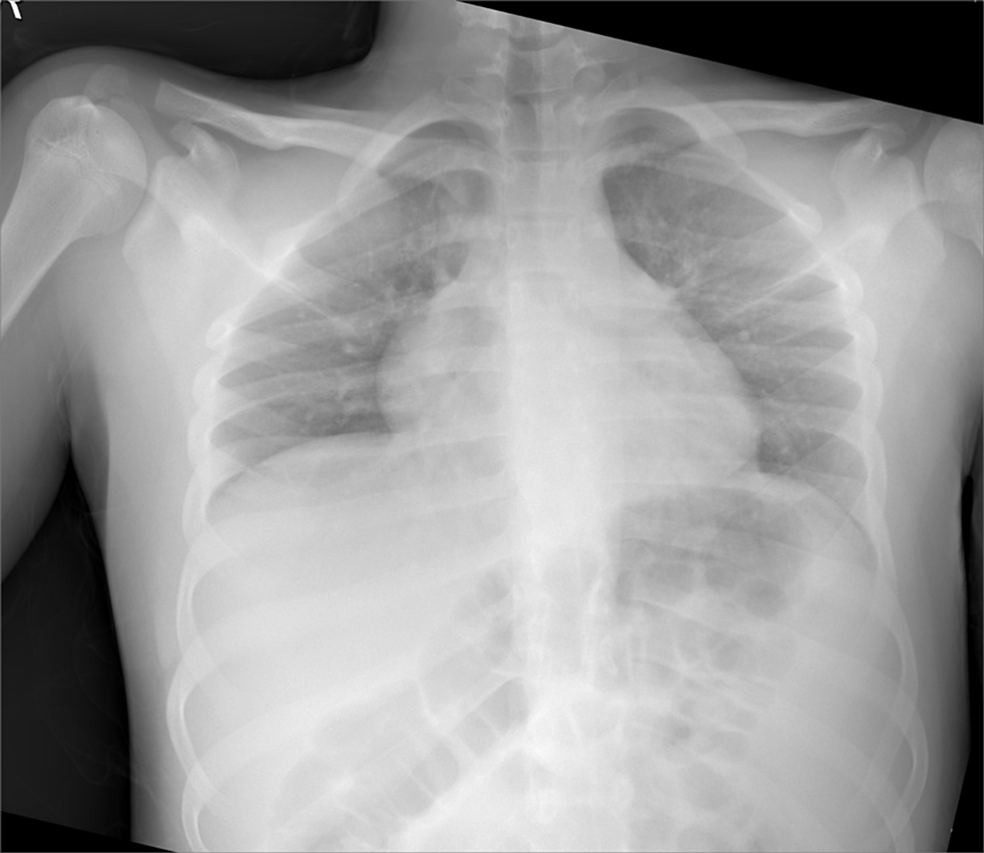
Chest x-rays revealed severe SARS-CoV-2-induced ARDS. ARDS - adult respiratory distress syndrome
